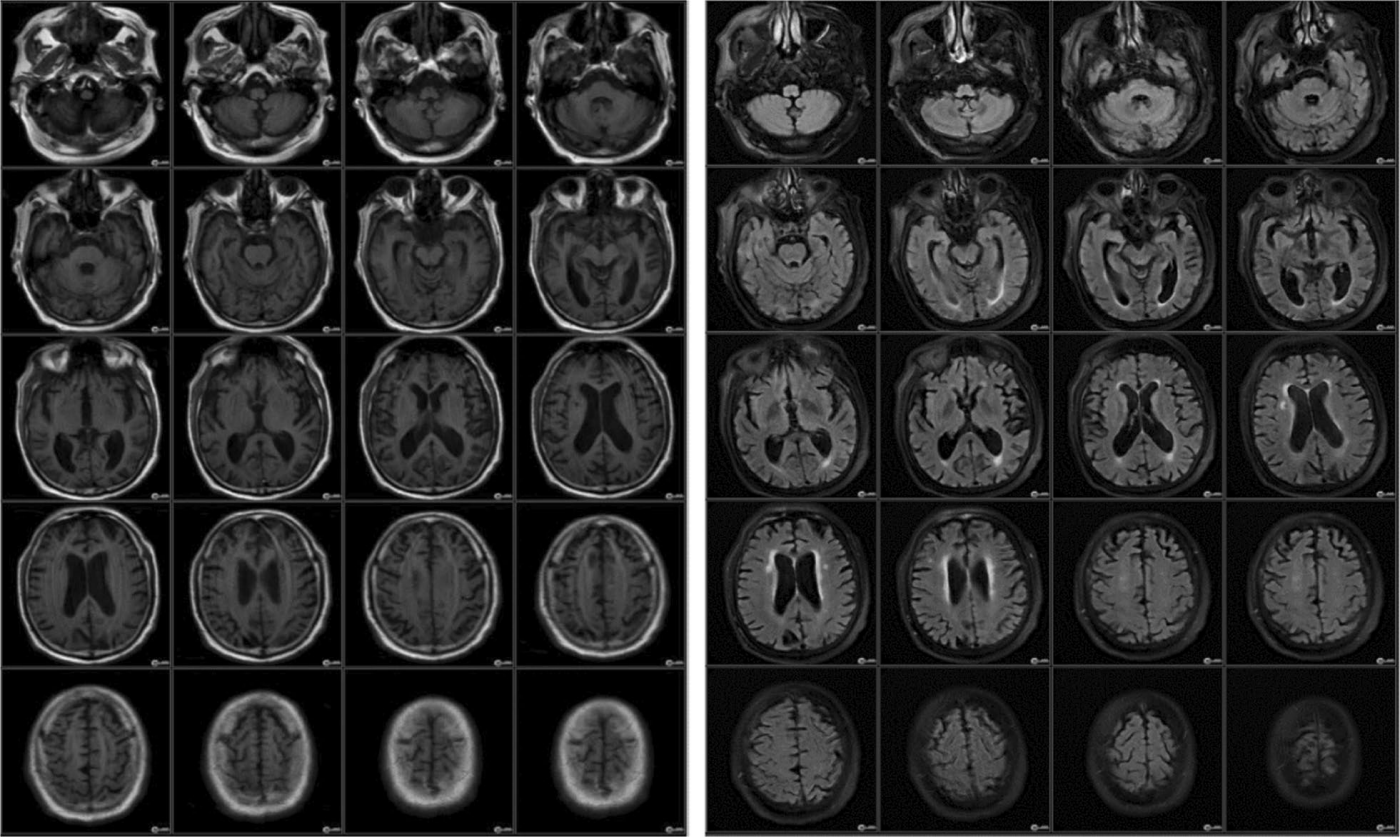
MRI brain axial T1 and FLAIR showing mild periventricular leukoencephalopathy, bilateral fronto-parietal small infarcts and central and to less extant cortical cerebral involutional changes
